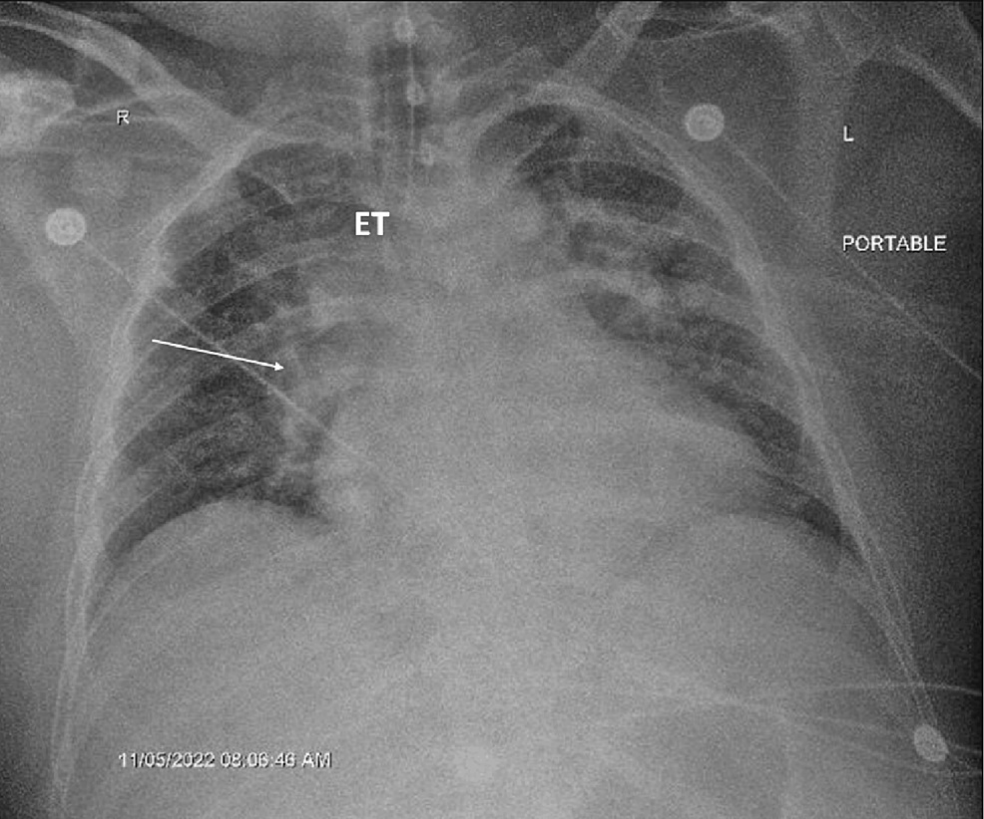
Chest X-ray showing slight engorgement of the pulmonary vasculature (arrow) and the endotracheal tube (ET) tip located in the lower trachea after the patient’s intubation in the post-anesthesia care unit
